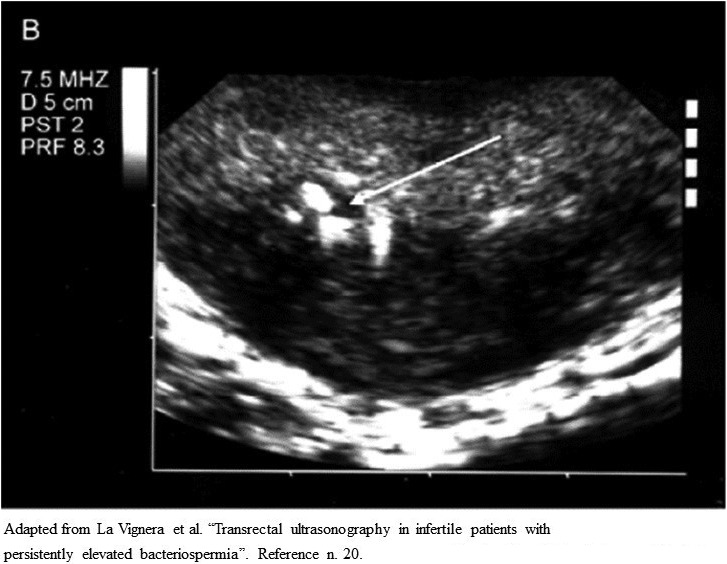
Ultrasound imaging of prostate in patients with persistent bacteriospermia
